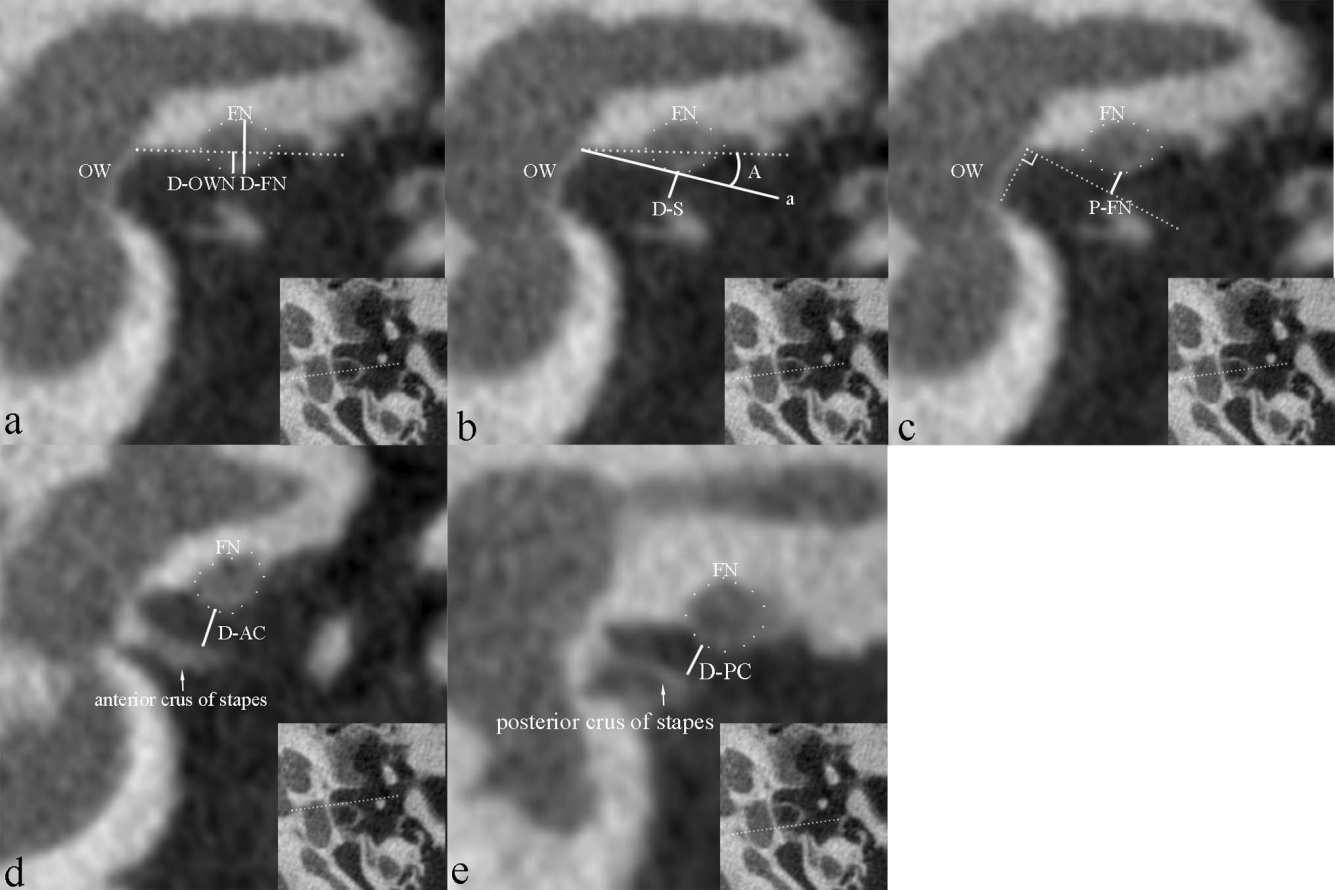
Position measurement of the facial nerve (FN) near oval window on U-HRCT standard coronal images. a The image passing through the middle of the stapes footplate; D-FN and D-OWN are measured. The dotted line is the upper border of oval window niche. b The same section as a, A is measured. Line a is the line connecting the lower margin of FN and the upper edge of oval window; dotted line is the upper border of oval window niche. D-S is also measured. c The same section as a, P-FN is measured. d The image passing through the anterior crus of stapes, D-AC is measured. e The image passing through the posterior crus of stapes, D-PC is measured. *OW* oval window, *FN* facial nerve
